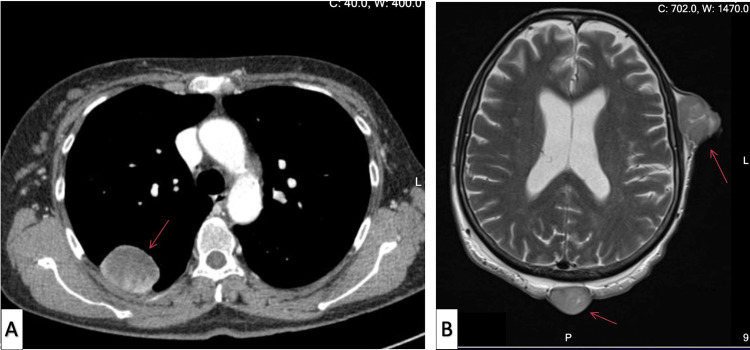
(A) CT of the chest shows a 3 cm mass in the right lower lobe and two nodules in the left lung. (B) MRI of the brain shows multiple well-circumscribed mass lesions overlying the left scalp.
